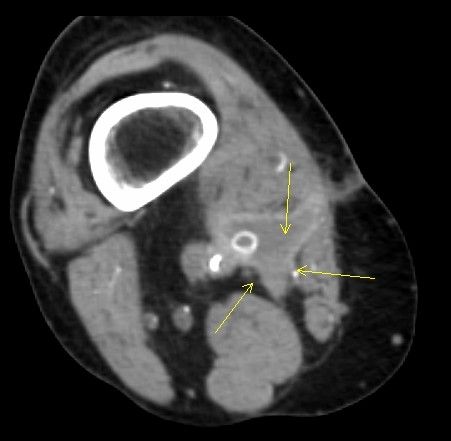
CT angiogram of a small seroma in the right popliteal fossa taken prior to seroma biopsy and removal of the femoral-popliteal ePTFE bypass graft. Arrows point to the location of the seroma.
